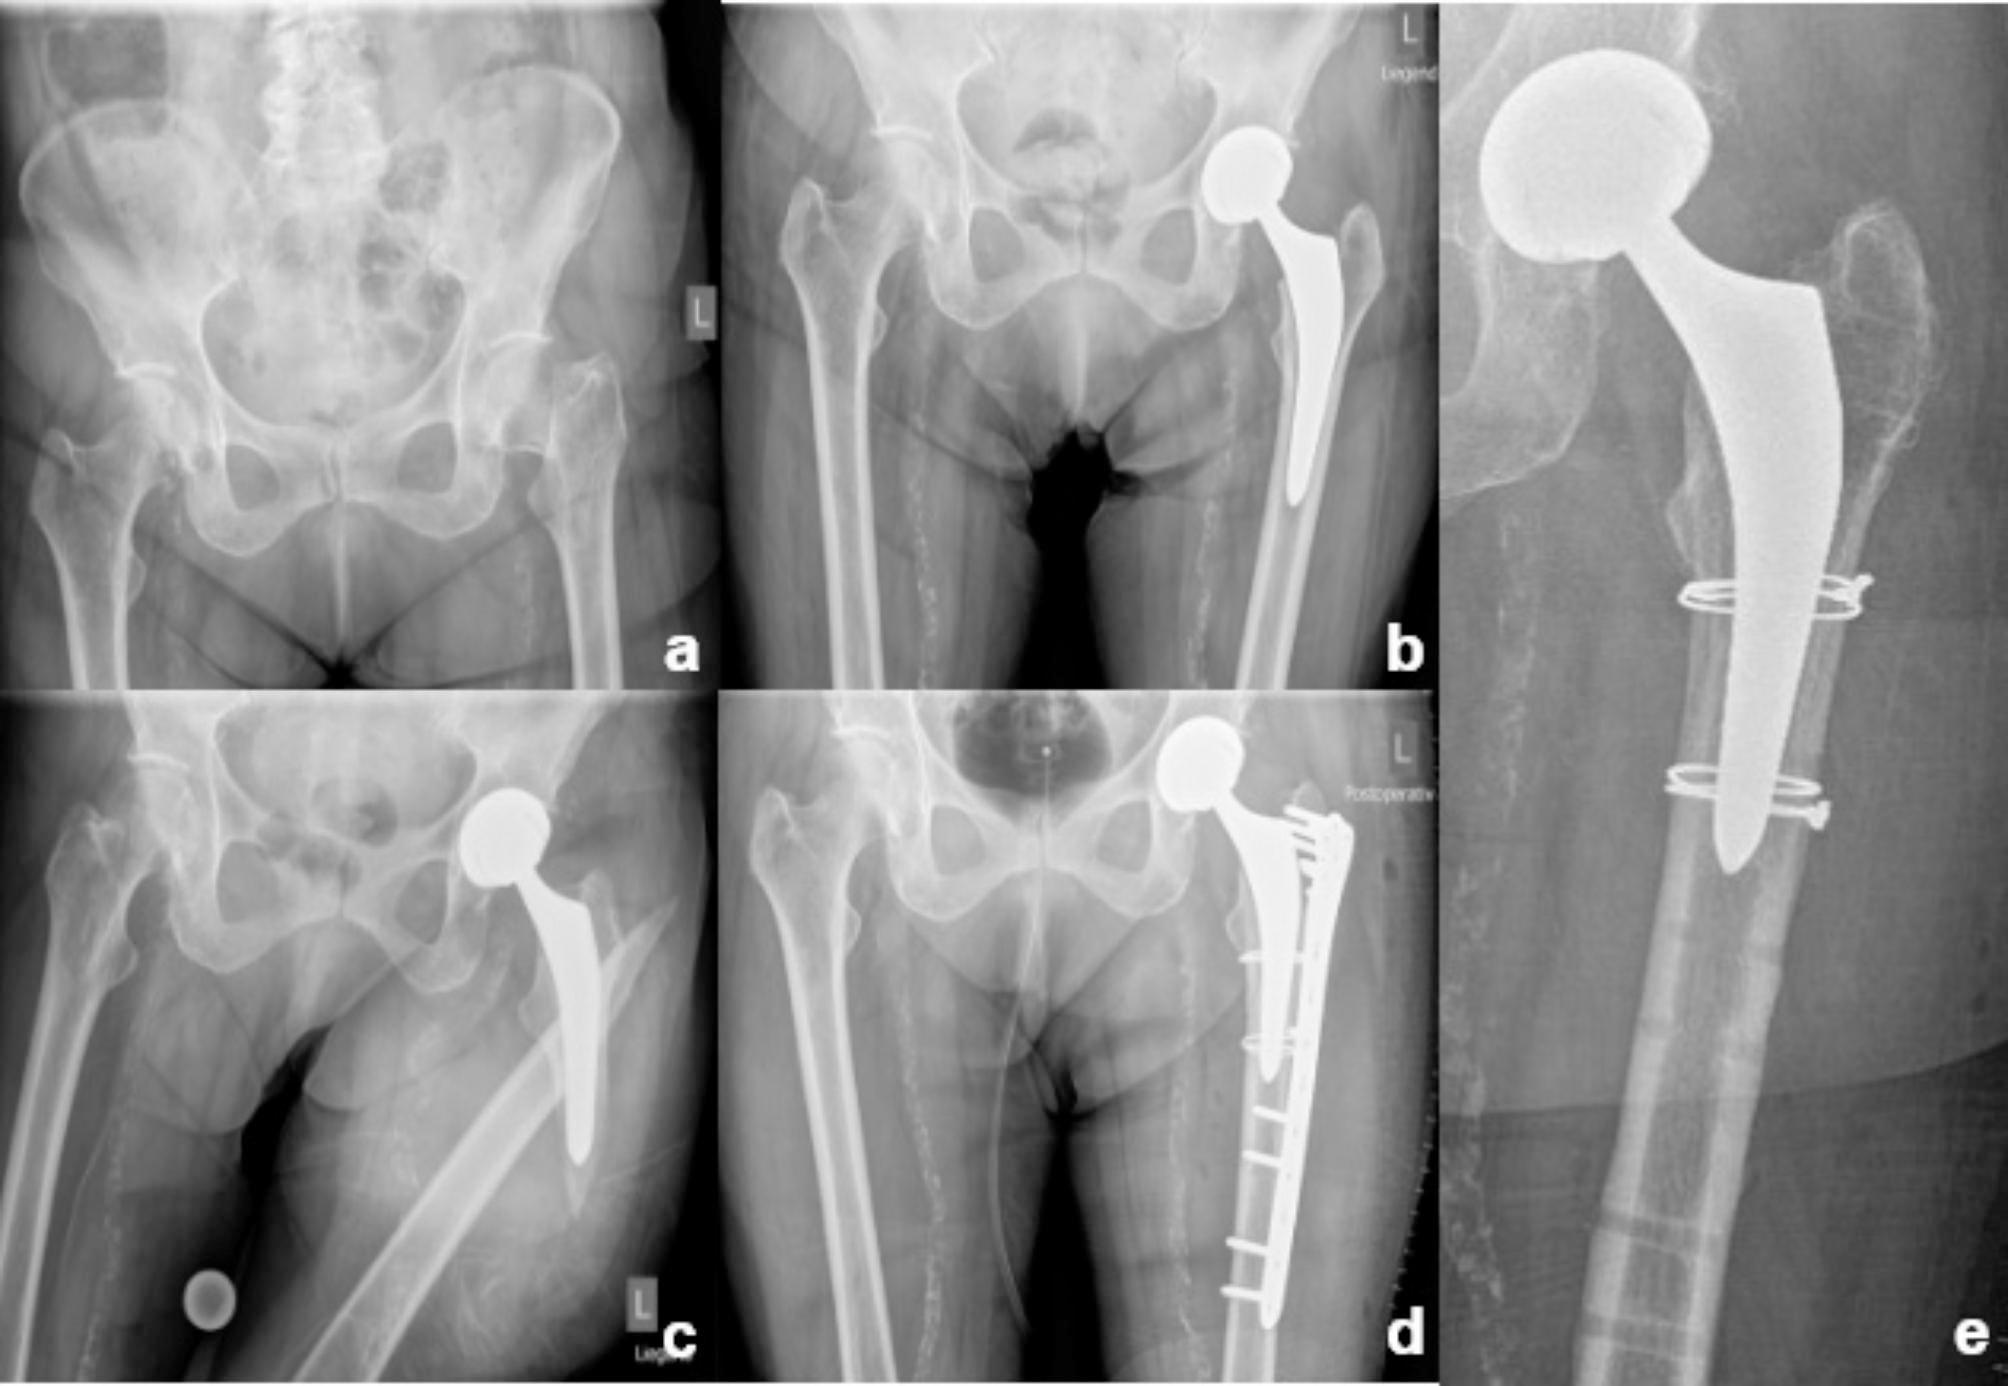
Conventional x-rays; (a) displaced femoral neck fracture of the left side. (b) treatment with uncemented hip hemiarthroplasty, (c) periprosthetic fracture (Vancouver B2), (d) osteosynthesis with reversed contralateral LISS plate and wire cerclages, (e) after removal of the plate
